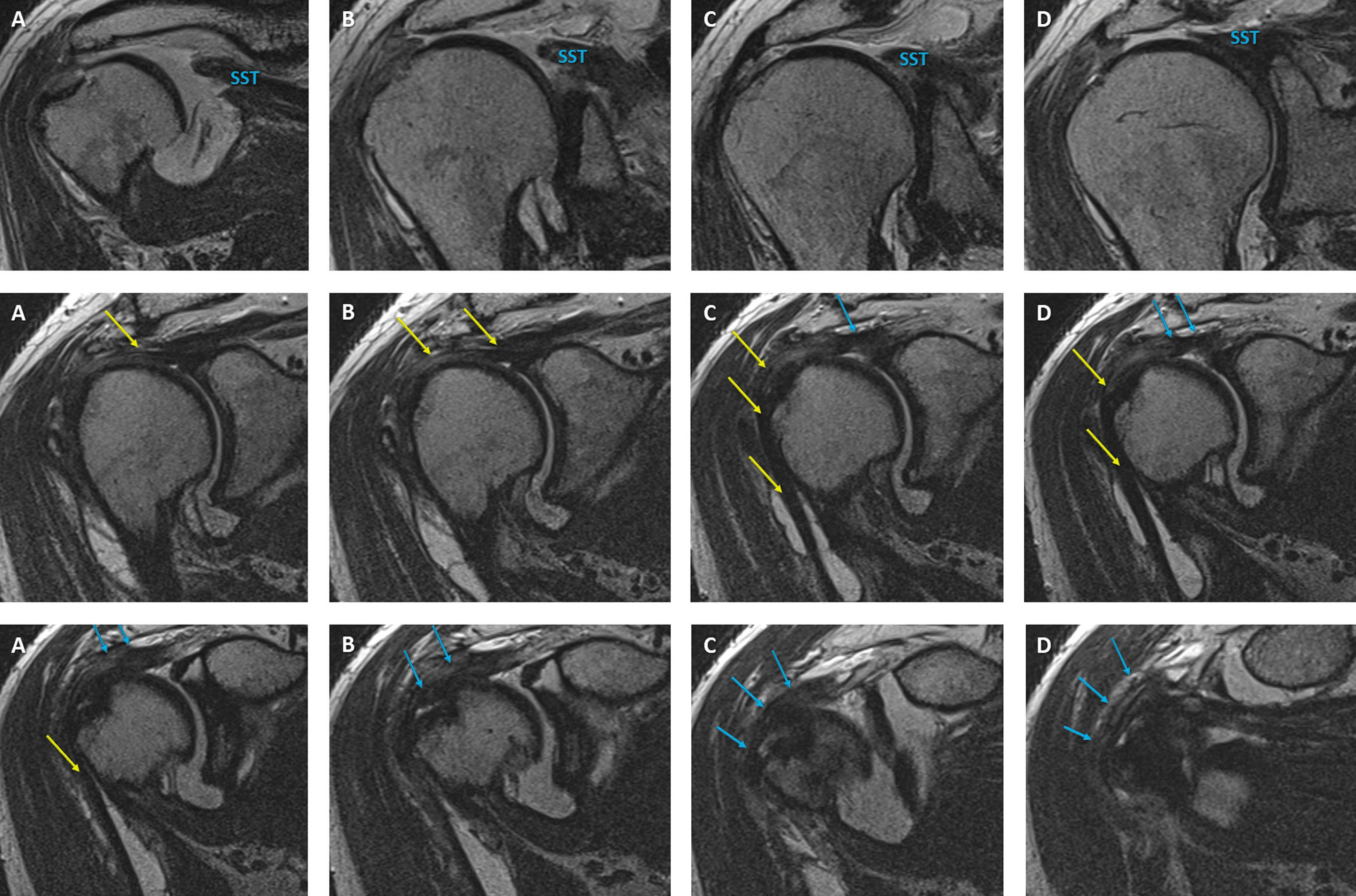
Sequential coronal oblique TSE T2-weighted MR arthrogram sections Consecutive coronal oblique turbo spin echo (TSE) T2-weighted MR arthrogram slices demonstrate complete rupture of the supraspinatus tendon (SST) and aponeurotic expansion of the supraspinatus tendon (blue arrows). Yellow arrows indicate the long head of the biceps brachii tendon.
